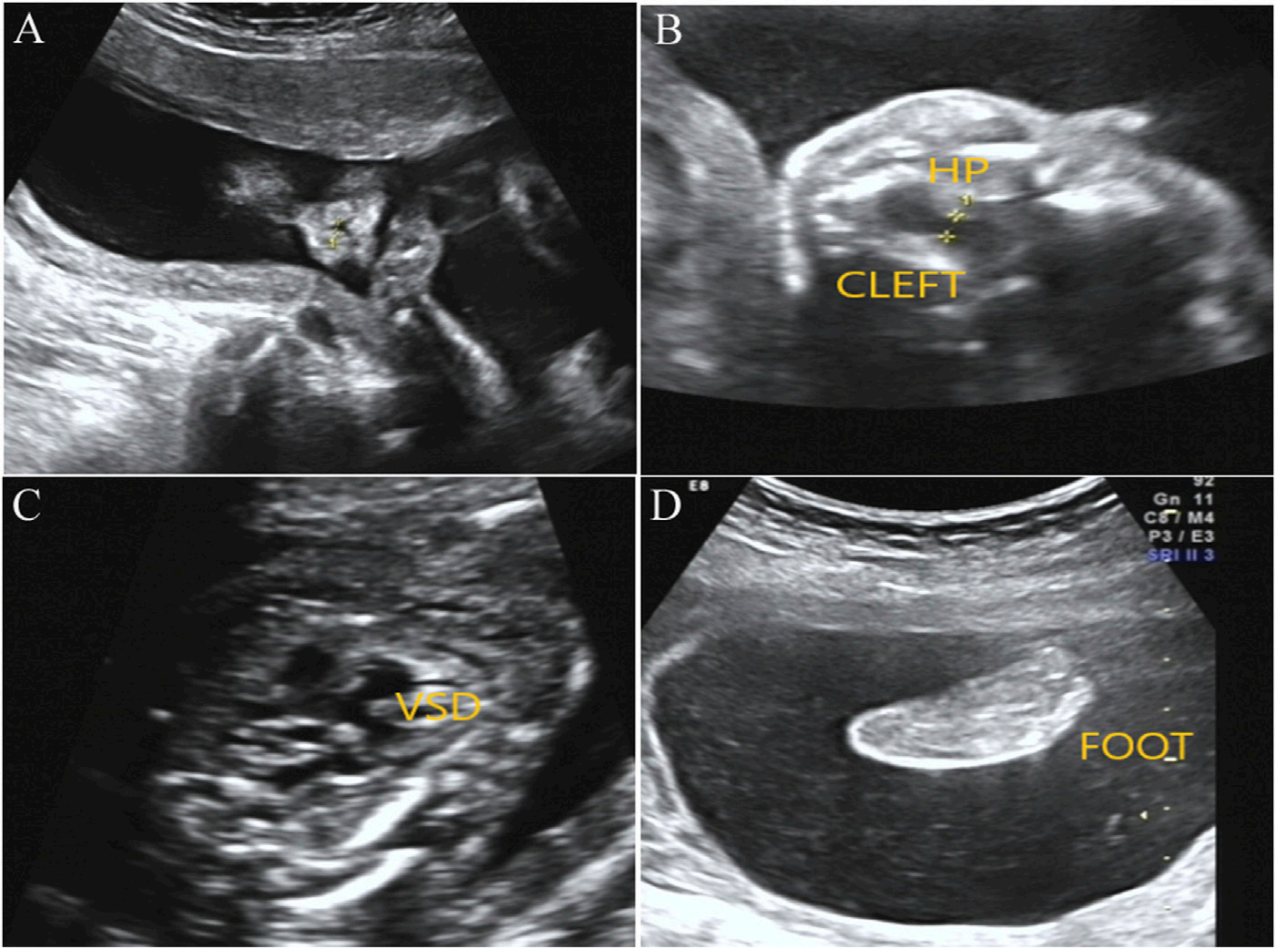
Prenatal ultrasound examination results in the fetus with *BOCR* variant. (A) Ultrasound detection results showed right alar fissure in the fetus. (B) Fetal ultrasound results indicated continuous interruption of cleft palate. HP, hard palate. (C) Fetal ultrasound results elicited fetal ventricular septal defect (VSD). (D) Toe syndactyly was also observed in the fetus by ultrasound examination.
